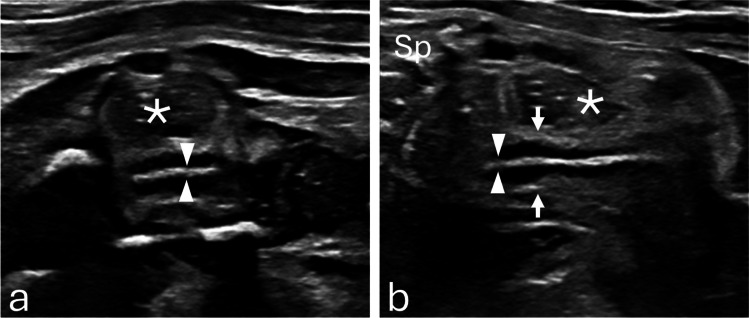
a Transverse and (b) sagittal grayscale ultrasound images at 29 weeks 4 days show the uterus (arrows) and non-thickened endometrium (arrowheads). The rectum (asterisk) lies posterior to the uterus. The fetal spine (Sp) is oriented anteriorly for image optimization. Thickness was measured as the maximal anteroposterior dimension of the echogenic endometrium, outer-to-outer between the anterior and posterior basal echogenic borders
